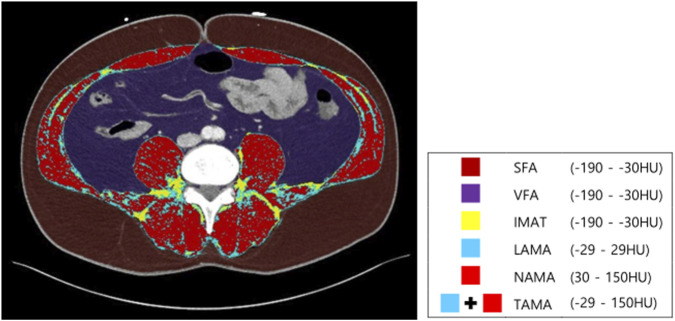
Abdominal body composition and muscle quality map generated from abdominopelvic computed tomography at the inferior endplate of L3 vertebra. HU, Hounsfield unit; SFA, subcutaneous fat area; VFA, visceral fat area; IMAT, intermuscular adipose tissue; LAMA, low-attenuation abdominal muscle area; NAMA, normal-attenuation muscle area; TAMA, total abdominal muscle area.
